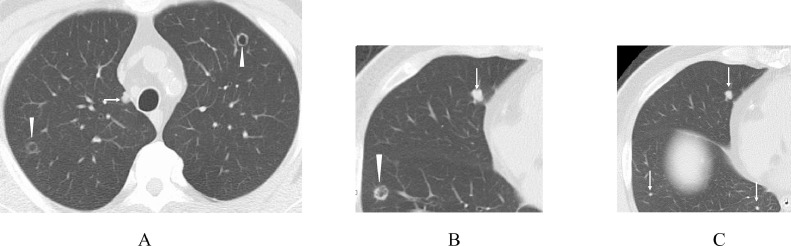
Thoracic computed tomography. (A) Two cavity-forming lesions with a thin and smooth cavity wall (arrowheads) and one solid lesion (arrow) were observed in the lungs. (B) One cavity-forming lesion with a relatively irregular wall (arrowhead) and a solid nodule (arrow) were observed. (C) Three solid lesions (arrows) were observed.
